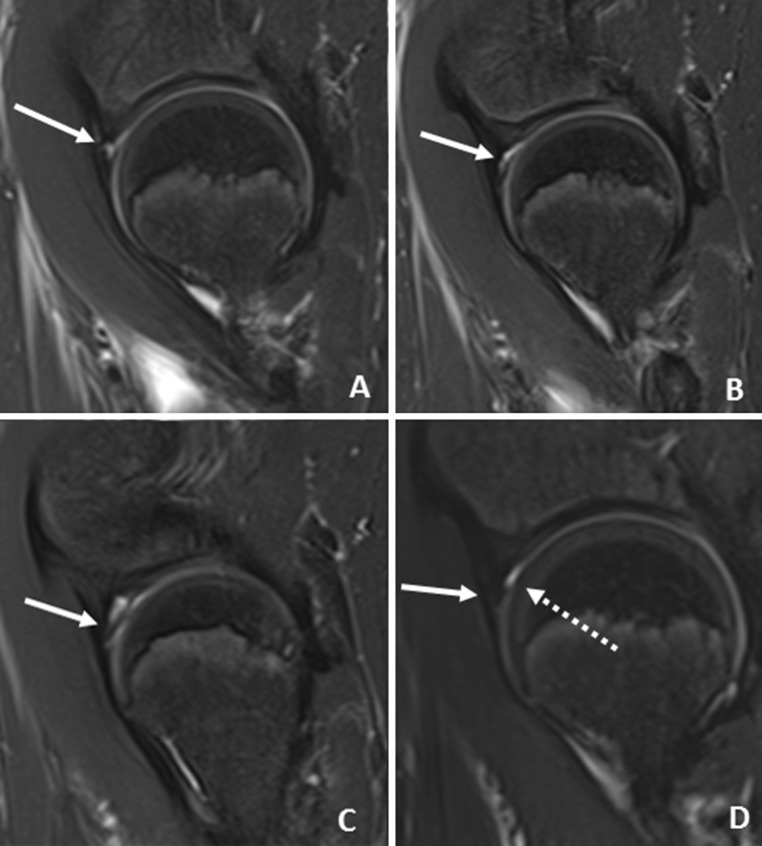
Consecutive sagittal T2-weighted fat-saturated images of an 11-year-old boy who underwent MRI of the pelvis for possible inflammatory bowel disease. a
